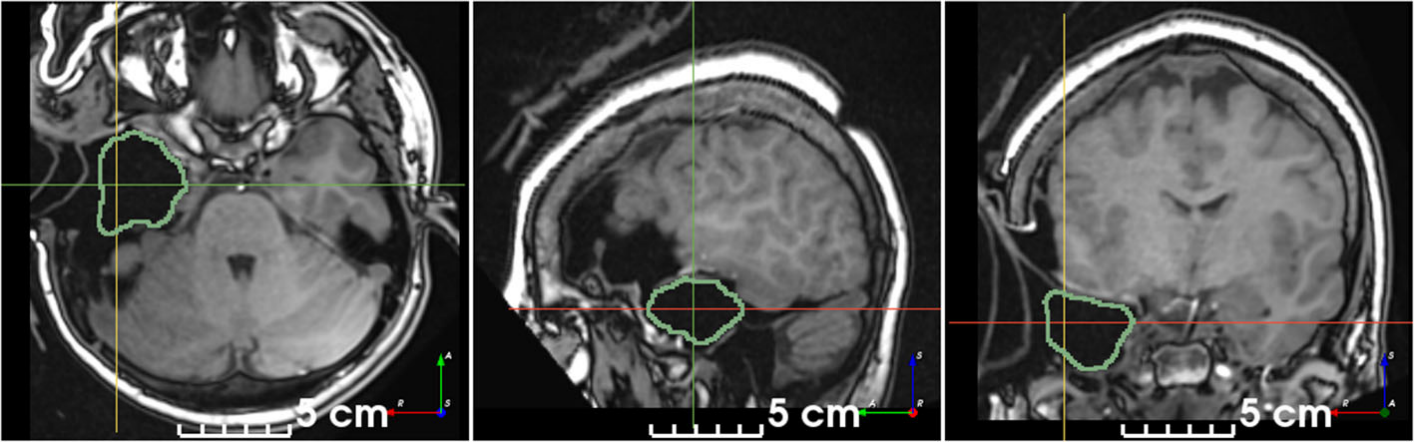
Qualitative result on an intraoperative MRI. The baseline model correctly discarded regions filled with air or CSF outside of the RC
